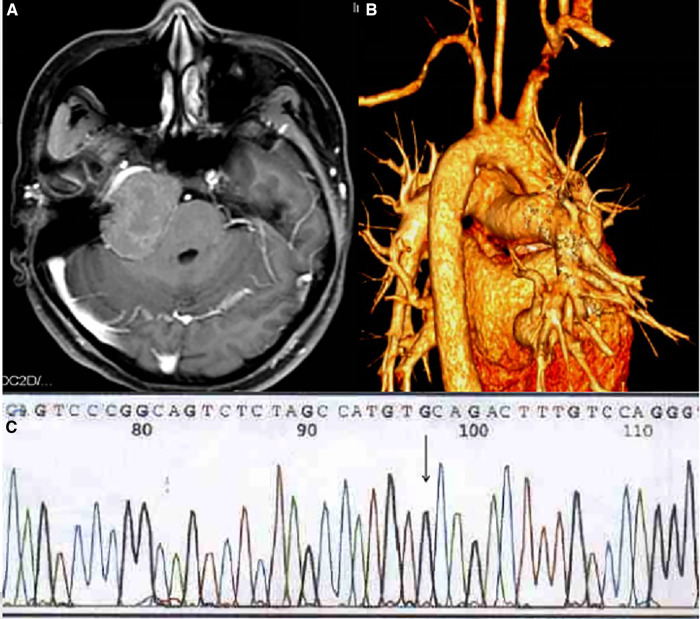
(A) MRI showd the skull base has long T1 and long T2 lesions on the right side of the middle straddle, with flair showing mixed signals; local bone destruction in the lesion. (B) Coronary CTA showed multiple mural thrombus with calcification in the proximal segment of the aortic arch and the three branches of the superior arch, moderate stenosis at the origin of the left common carotid artery. (C) T-G mutation at position 97 in exon 6 of LDL-R gene (arrow).
